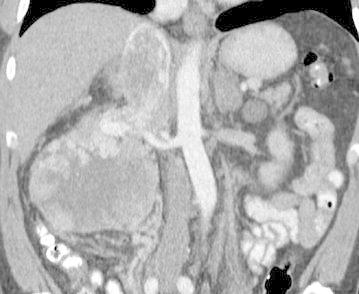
CT scan showing a large right renal cancer with IVC thrombus above hepatic veins but below diaphragm (IIIc)
